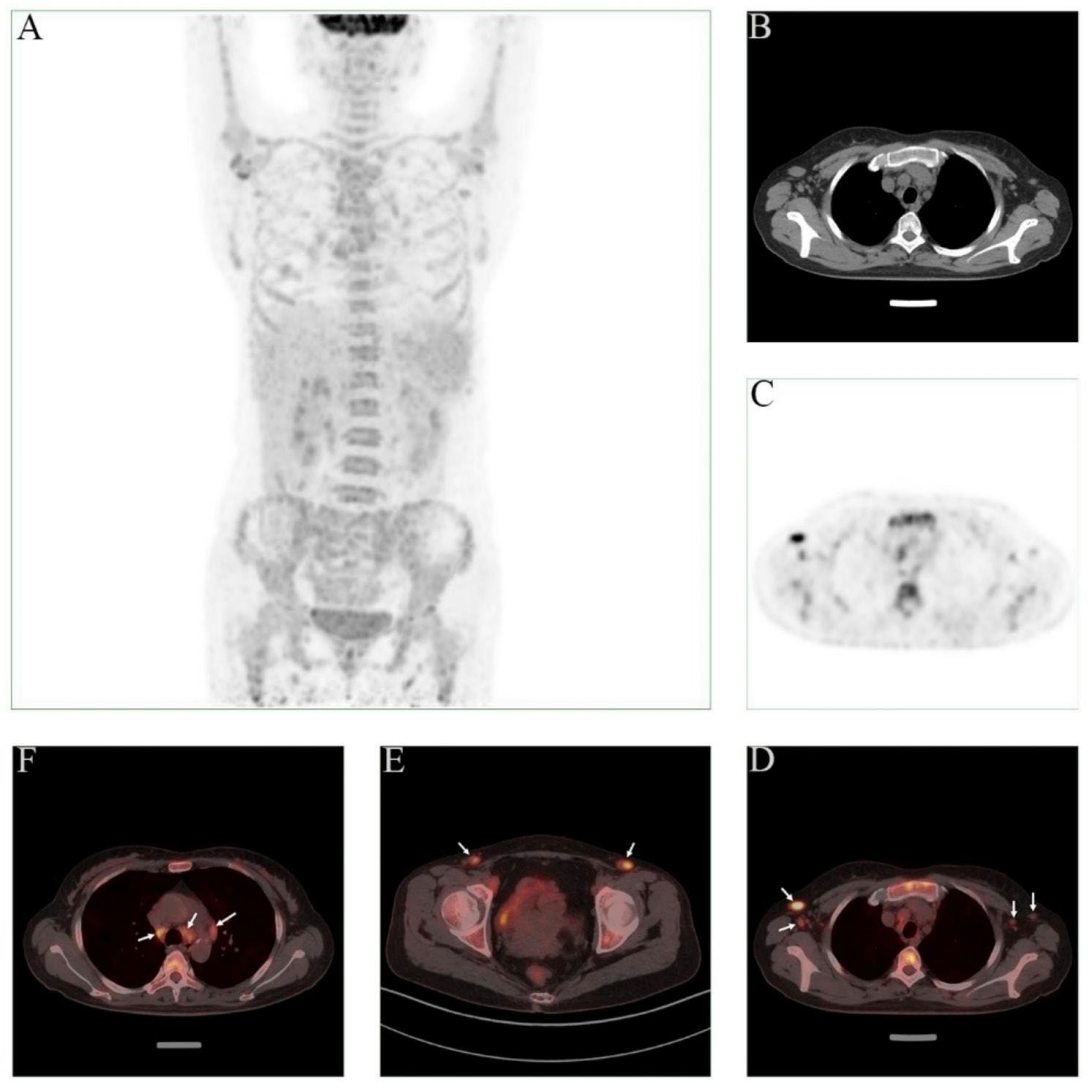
(A) ^18^ F-FDG PET/CT images showing diffuse elevated ^18^ F-FDG metabolism in bone and multiple lymph nodes throughout the body; (B-D) PET/CT images of axillary lymph nodes showed high uptake of 18 F-FDG, suggesting increased metabolism; (E, F) Bilateral mediastinal pleura, and inguinal lymph nodes all showed increased metabolism
